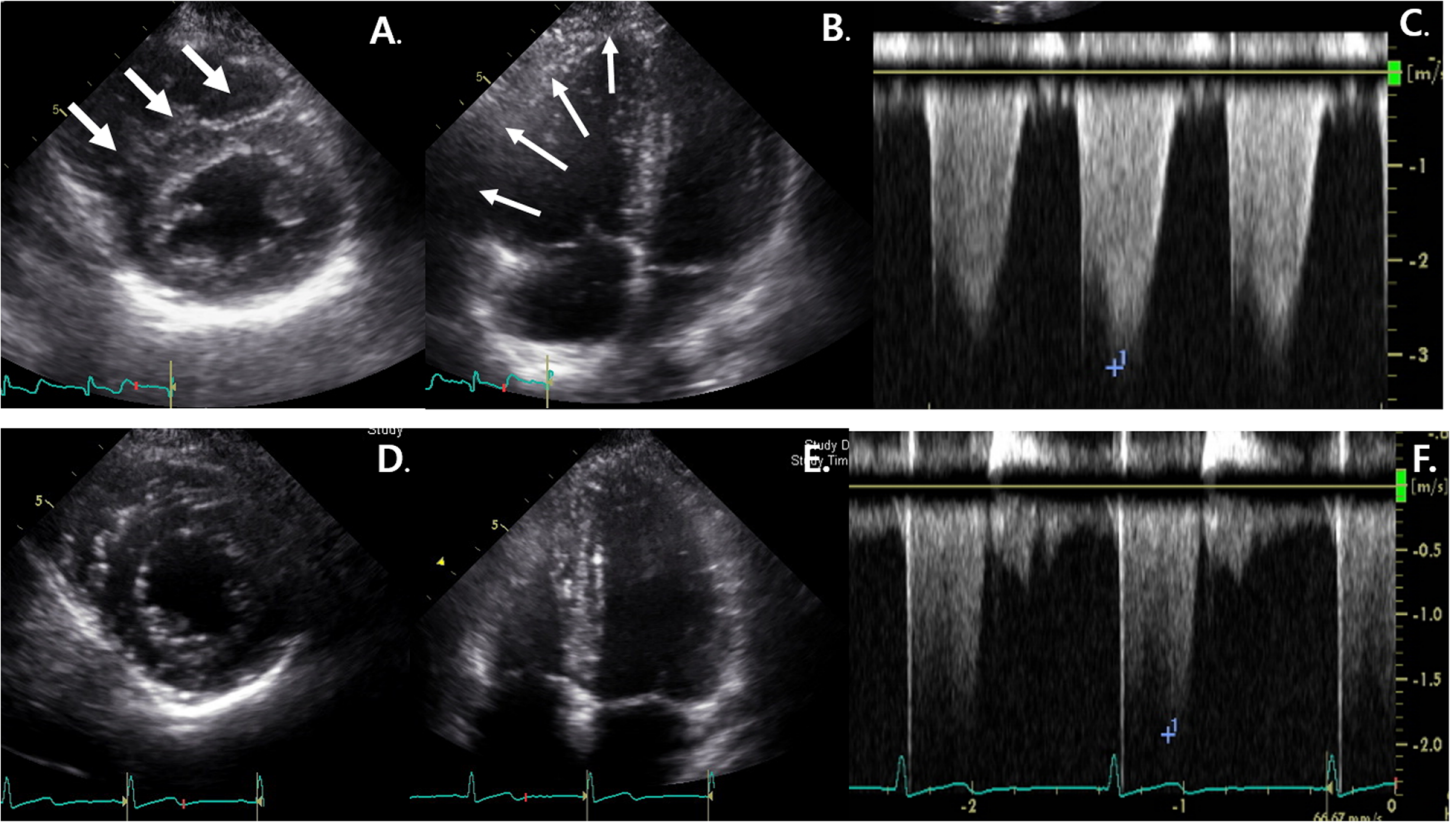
Transthoracic echocardiographic (TTE) findings. a Parasternal short axis view on TTE performed on admission showing flattening of the interventricular septum (D-shaped left ventricle (LV): White Arrow). b Apical-four-chamber view on TTE performed on admission showed marked right ventricular (RV) dilatation (White Arrow). c Continuous wave Doppler echocardiographic study performed on admission. Pulmonary artery systolic pressure (64 mmHg) was calculated using maximum velocity of the tricuspid regurgitation jet (Vmax = 3.3 m/sec) and estimated right atrial pressure (20 mmHg). d Parasternal short axis view on TTE performed 3 months after anticoagulation therapy showing normalization of RV size and disappearance of the D-shaped LV. e Repeated TTE performed 3 months after anticoagulation therapy showed normalization of RV cavity with improved systolic function. f Continuous wave Doppler echocardiographic study performed 3 months after anticoagulation therapy. Pulmonary artery systolic pressure was normalized using maximum velocity of the tricuspid regurgitation jet (Vmax = 1.9 m/sec)
